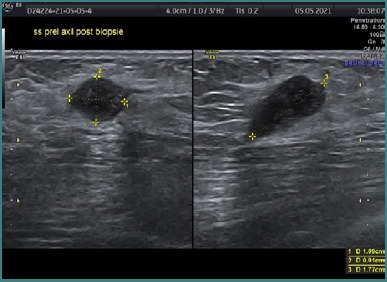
Post-biopsy ultrasound assessment shows a flattened image with observed slight extravasation of dense content upon withdrawal of the puncture needle
